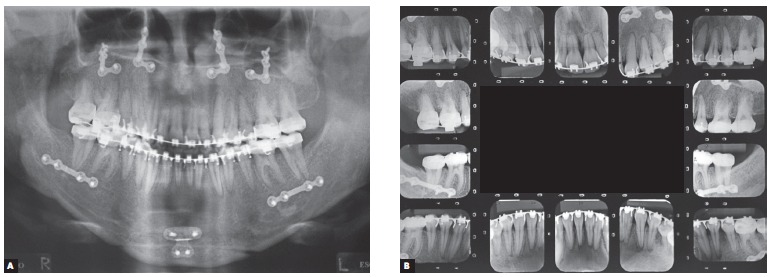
Final panoramic (A) and periapical (B) photographs.
